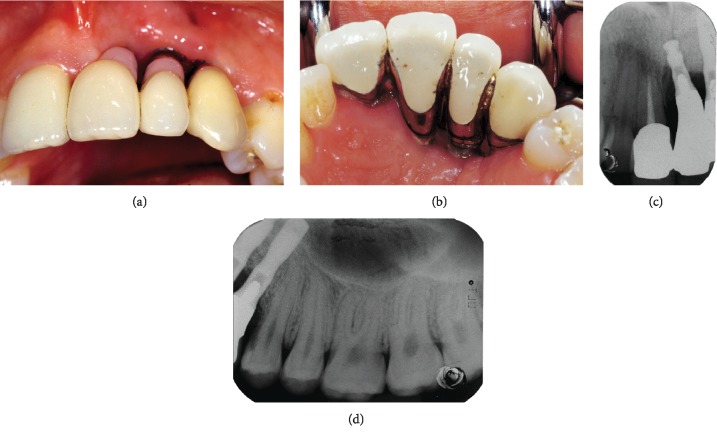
Preoperative clinical and radiographic views: (a) buccal aspect, (b) palatal aspect, (c) periapical radiograph of the right central incisor, (d) periapical radiograph of the left first premolar.
